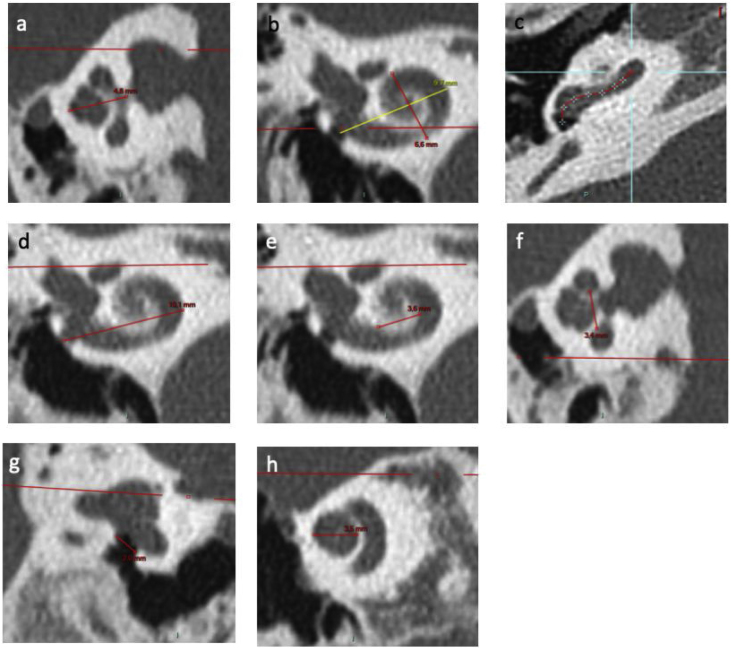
Inner ear CT scan measurements. (a) Height of cochlea on an axial view; (b) Measurements of length A on a reconstruction through modiolus. B measurement corresponds to width of cochlea; (c) Identification of points in basal turn of cochlea via the application “vessel analysis” to measure length of unrolled cochlea; (d) External diameter of basal turn of cochlea on a frontal view; (e) Internal diameter of basal turn of cochlea on a frontal view; (f) Internal diameter of basal turn of cochlea on a sagittal view; (g) RW diameter on a reconstruction from Saylisoy et al.^16^; (h) Diameter of apical turn of cochlea.2)the width of cochlea (Fig. 2b) was quantified perpendicular to “A”, defined as the longest measurement between the round window (RW) and the lateral wall of cochlea through the modiolus,3)the length of cochlea (Fig. 2c) was quantified by “unrolling” the cochlea using the “vessels analysis” tool in the PACS MIRC Carestream software and measuring the mean value between the central and external lumen. The length of cochlea on 720° was calculated according to previous studies^15^ (length = 3.65*A - 3.63),4)the diameter of external basal turn (Fig. 2d) was quantified in terms of the mean value between the axial and sagittal plane of the longest measurement between the RW and the lateral wall of basal turn,5)the diameter of the internal basal turn was quantified in terms of the mean value between the frontal (Fig. 2e) and sagittal planes (Fig. 2f) of the longest measurement between medial wall of basal turn.6)the diameter of the RW (Fig. 2g) was quantified according to the strict plane described by Saylisoy et al.^16^,7)the diameter of apical turn (Fig. 2h) was quantified in terms of the distance between the lateral walls through the modiolus, on a plane through the apex,8)the number of spiral turns was calculated from the RW to apex, with one turn counted each time the spiral passed through the RW plane, and9)the volume of cochlea was quantified using 3D-Doctor (version 3.5; Able Software Corp., Lexington, MA, USA) after interactive segmentation of cochlea.
